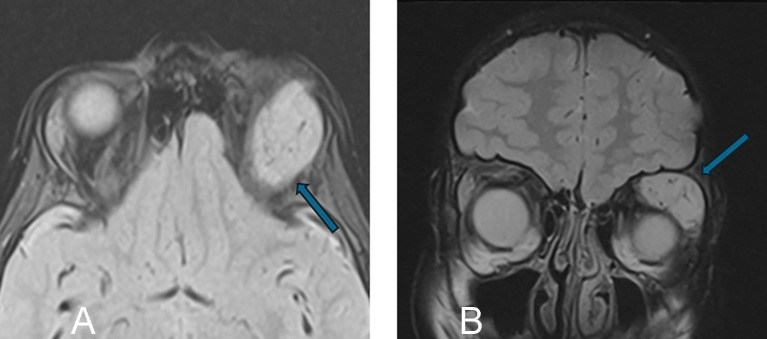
MRI images in an 8-year-old boy with embryonal RMS. MRI imaging is preferred, given the desire to avoid any radiation in children. However, CT scans are readily available and do not require sedation in young children. (A, B) Axial and coronal T2 MRI images show a large mass involving the left lacrimal gland (arrow).
